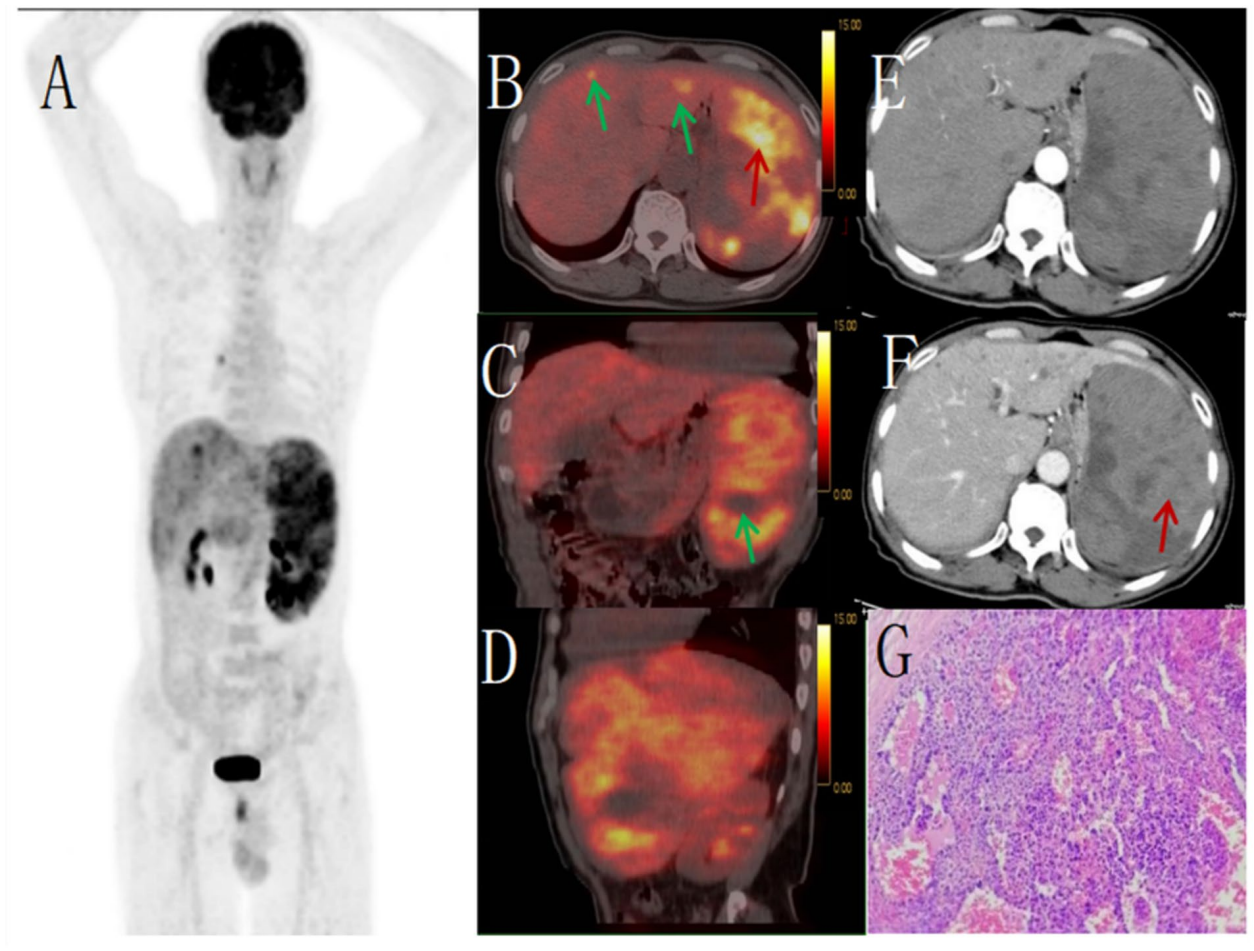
Male, 67 years old, primary splenic angiosarcoma. (A) whole body MIP, (B) axial fusion, (C) coronal fusion, (D) sagittal fusion, (E) enhanced CT-arterial phase, (F) enhanced CT-venous phase, (G) (H-E × 400). 18F-FDGPET/CT showed multiple nodular and massive lesions in the spleen with multiple necrotic areas, PET/CT images showed an abnormal increase in diffuse and uneven uptake of 18F-FDG in the spleen, with a SUVmax of 9.0 (red arrow, B) and no FDG uptake in some necrotic areas (green arrow, C). There were multiple nodular lesions in the liver, the level of FDG metabolism increased, the SUVmax was 5.6 (green arrow, B). Enhanced CT showed diffuse uneven mild enhancement of spleen, and the degree of enhancement increased with time (red arrow, F). Multiple nodular low-density shadows were seen in the liver, which were slightly enhanced by enhanced scan. PET/CT-guided splenic biopsy confirmed the diagnosis of primary splenic angiosarcoma (G).
